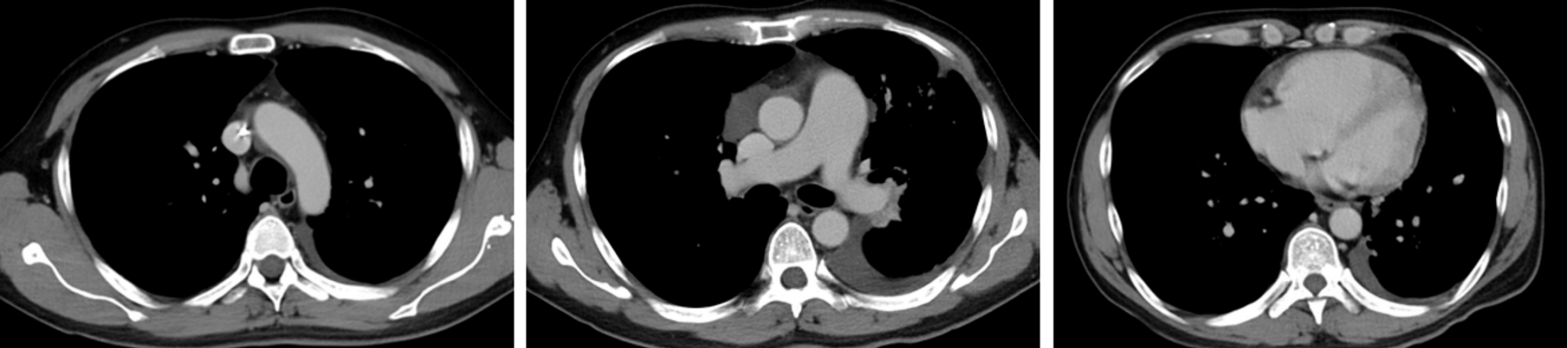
Contrast-enhanced chest computed tomography on admission. Contrast-enhanced chest computed tomography imaging showed no evidence of pulmonary embolism.
